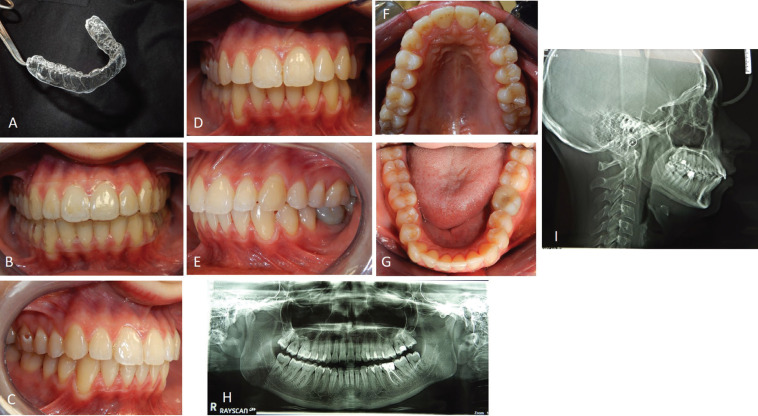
Appearance of a SORRIDI® clear aligner (A). The patient wearing both “SORRIDI” clear aligners (B). Post-treatment intraoral photos of the patient (C-G). Post-treatment radiographs of the patient (H, I).
